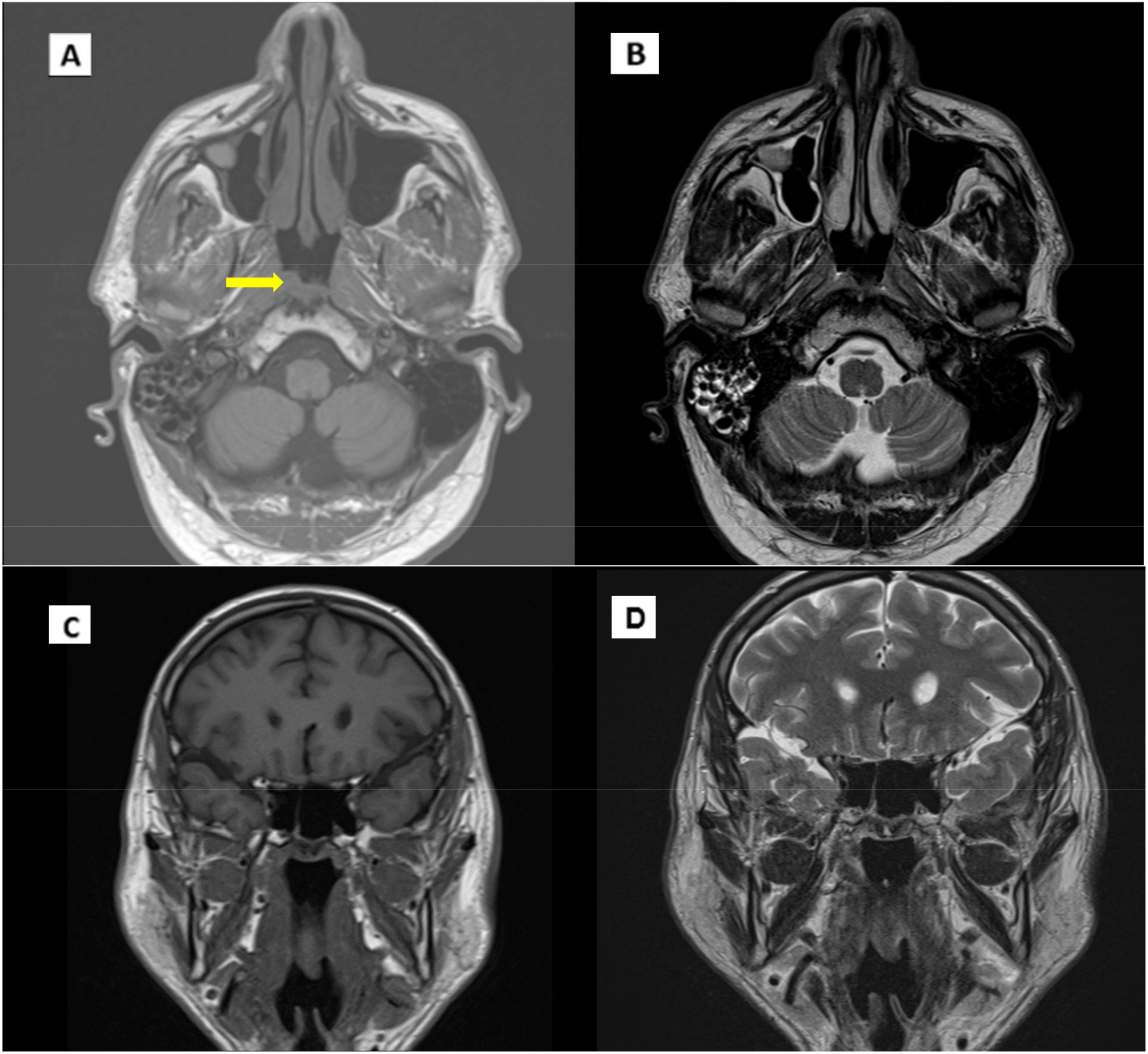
MRI scan in January 2023. (A) T1 Coronal scan. (B) T1 axial scan. (C) T2 coronal scan. (D) T2 axial scan. Arrow indicates pathological thickening of the nasopharynx.
